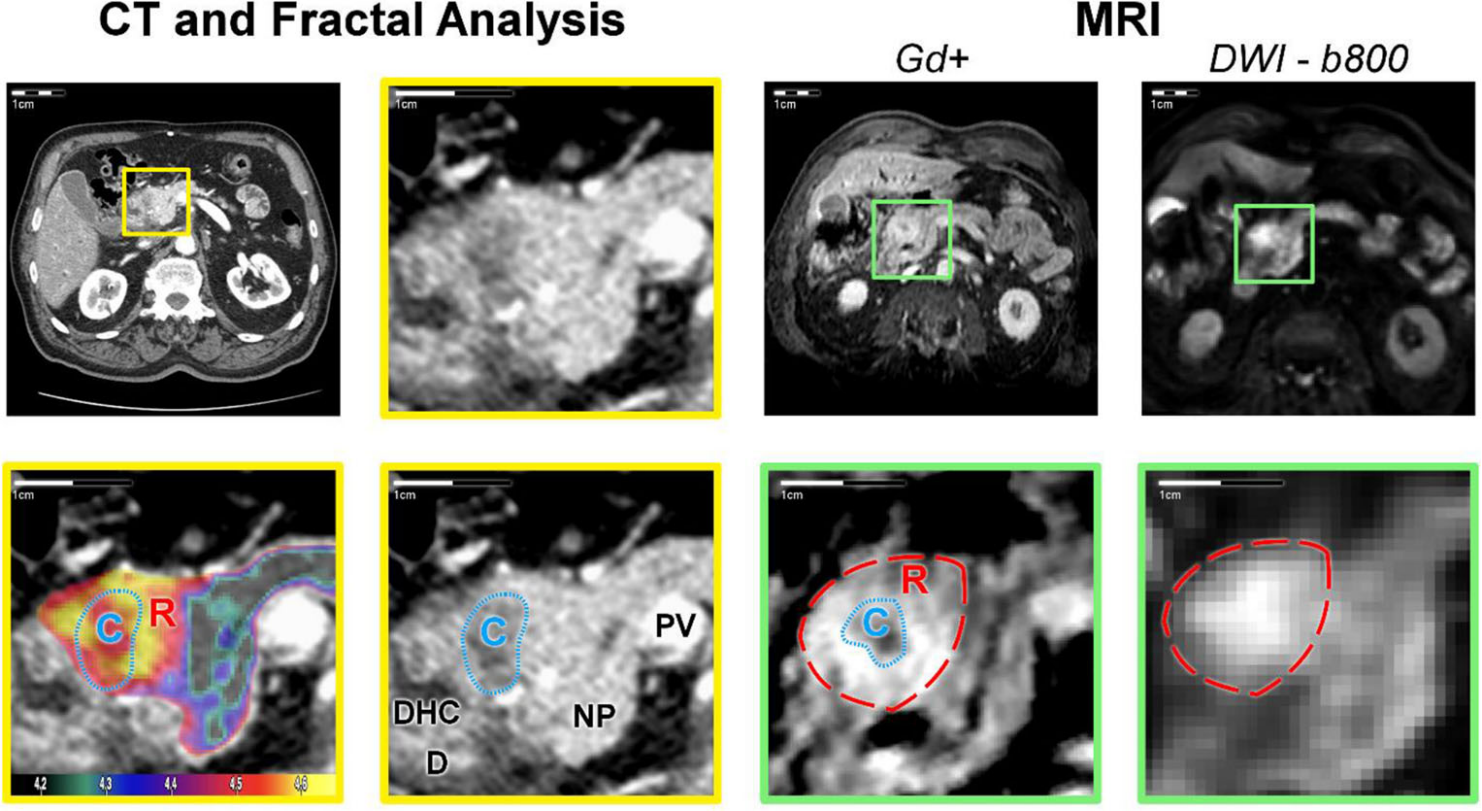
Representative example of pancreatic ductal adenocarcinoma in the pancreatic head. A para-axial reformation was chosen to simultaneously depict FD differences in the tumour core, rim, and adjacent non-tumourous pancreatic tissue. The panel organisation is analogue to Figure 1. On CT, the tumour core is the only visible portion of the tumour. In contrast, the tumour rim (R, high FD mapping values or red dashed line) is well depicted on MRI and FD maps and its perfusion pattern is more chaotic than in the tumour core and adjacent non-tumourous pancreatic tissue as indicated by the FD. Tumour volume was 3 ml on CT, 8 ml on FD map, and 9 ml on MRI. Tumour diameter was 18 mm on axial slices, whereas Feret’s calculated maximum diameter along the longest axis in arbitrary orientation was 20 mm on CT, 33 mm on FD map, and 29 mm on MRI. C, tumour core; R, tumour rim; NP, non-tumourous pancreatic tissue; PV, portal vein; D, duodenum; DHC, ductus hepato-choledochus
